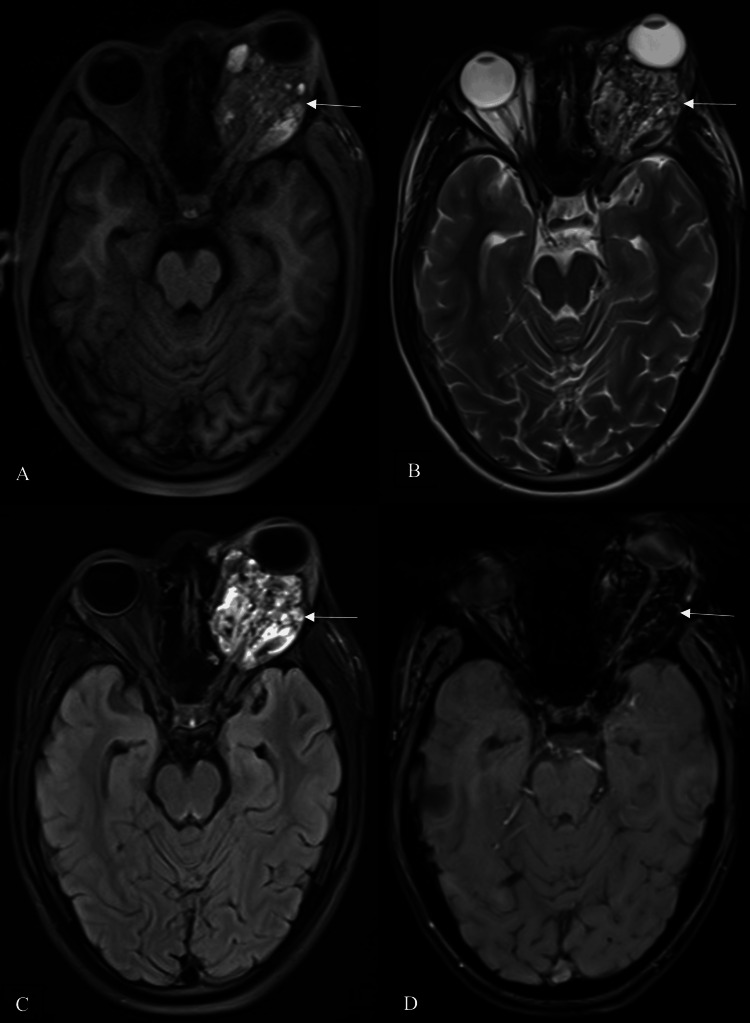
MRI of both orbits. A: T1WI, B: T2WI, C: FLAIR, D: GRE Axial images show an ill-defined, multi-locular heterogeneous solid-cystic mass in the left retrobulbar space. The lesion exhibits an isointense to hyperintense signal on T1WI, a hyperintense signal on T2WI and FLAIR sequences with fluid-fluid levels, and blooming on the GRE sequence MRI: Magnetic resonance imaging, FLAIR: Fluid attenuated inversion recovery, GRE: Gradient echo
